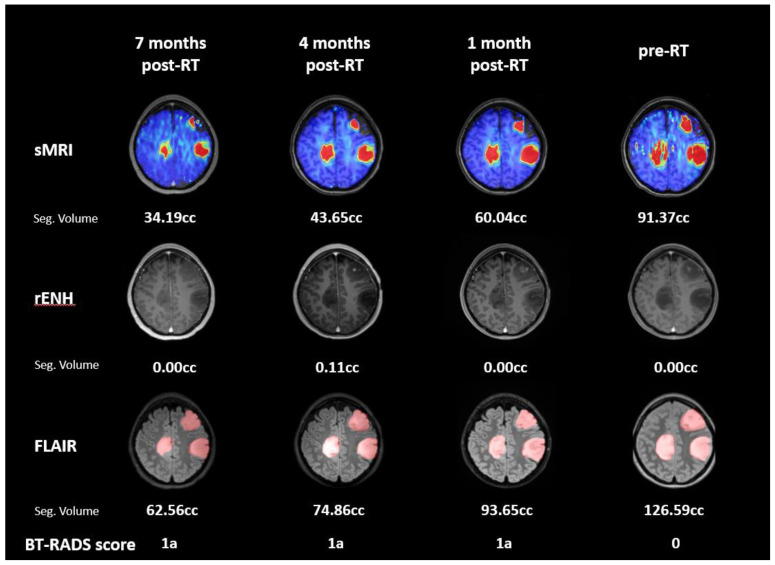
Subject 2 longitudinal follow-up imaging shown in BrICS-LIT. Four follow-up images and their corresponding sMRI, T1w-CE, and T2 FLAIR are shown chronologically from right to left, with the pre-RT treatment planning image first. Lesion volumes have been segmented, and each follow-up is scored by a neuroradiologist. Little to no contrast enhancing tumor was seen in follow-ups. The imaging shows a positive response to treatment with decreasing volumes on sMRI from pre-RT to 7 months post-RT; the FLAIR volumes also decreased during this time period. Each follow-up is scored as BT-RADS 1a corresponding to disease improvement.
